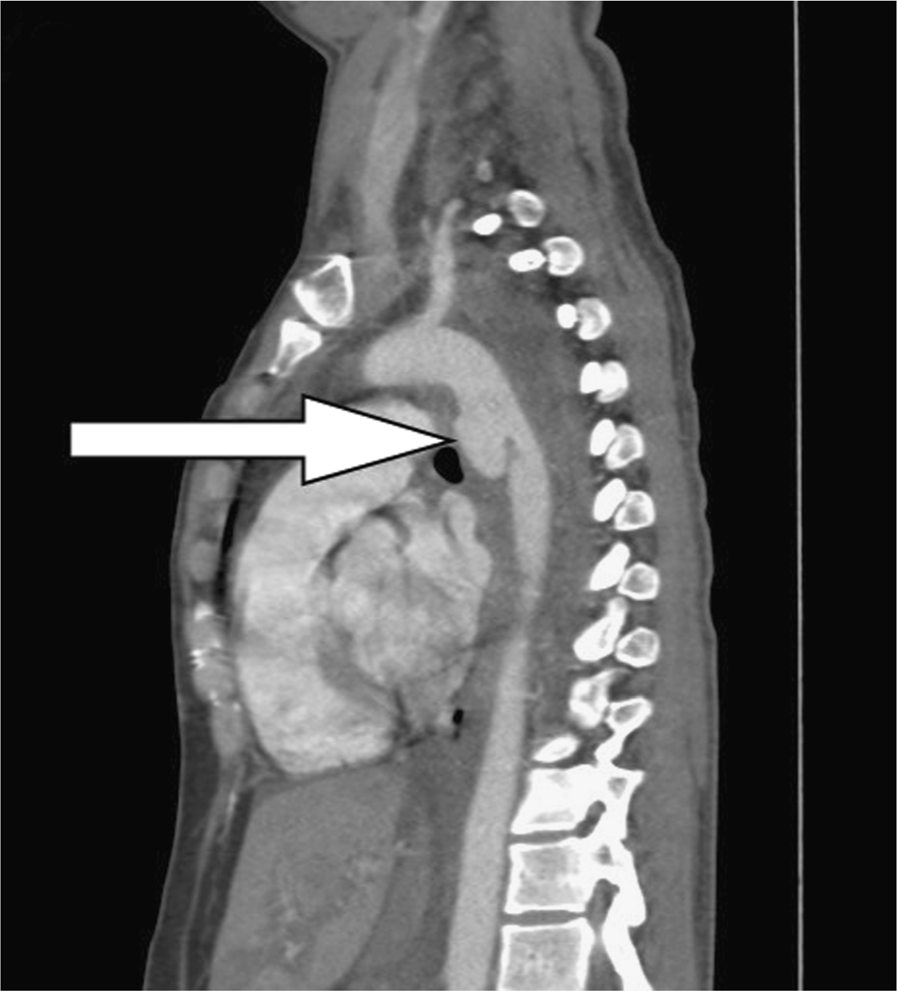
The coronal view of CTA depicted a thoracic aortic pseudoaneurysm (arrow) below the aortic arch, hemothorax and hemomediastinum.
